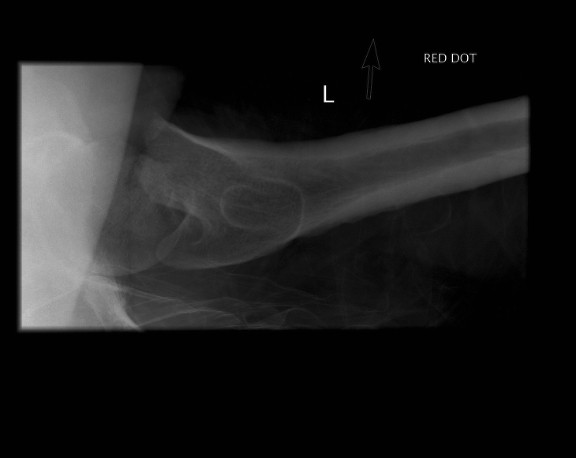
Radiograph showing lateral view of his fractured left proximal femur.
